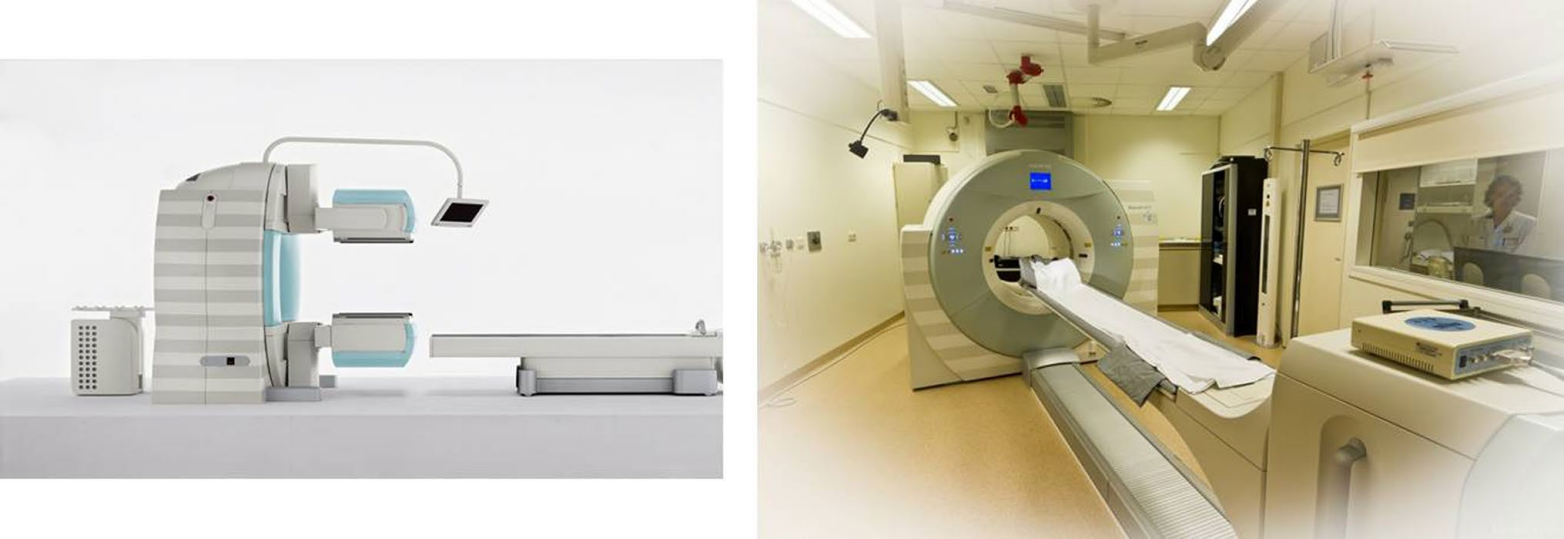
Left image* gamma camera with SPECT-CT possibility (Siemens Symbia T).* Right* PET-CT camera (Siemens Biograph mCT 64-slice). Image courtesy: Siemens Medical Systems, Knoxville, TN
